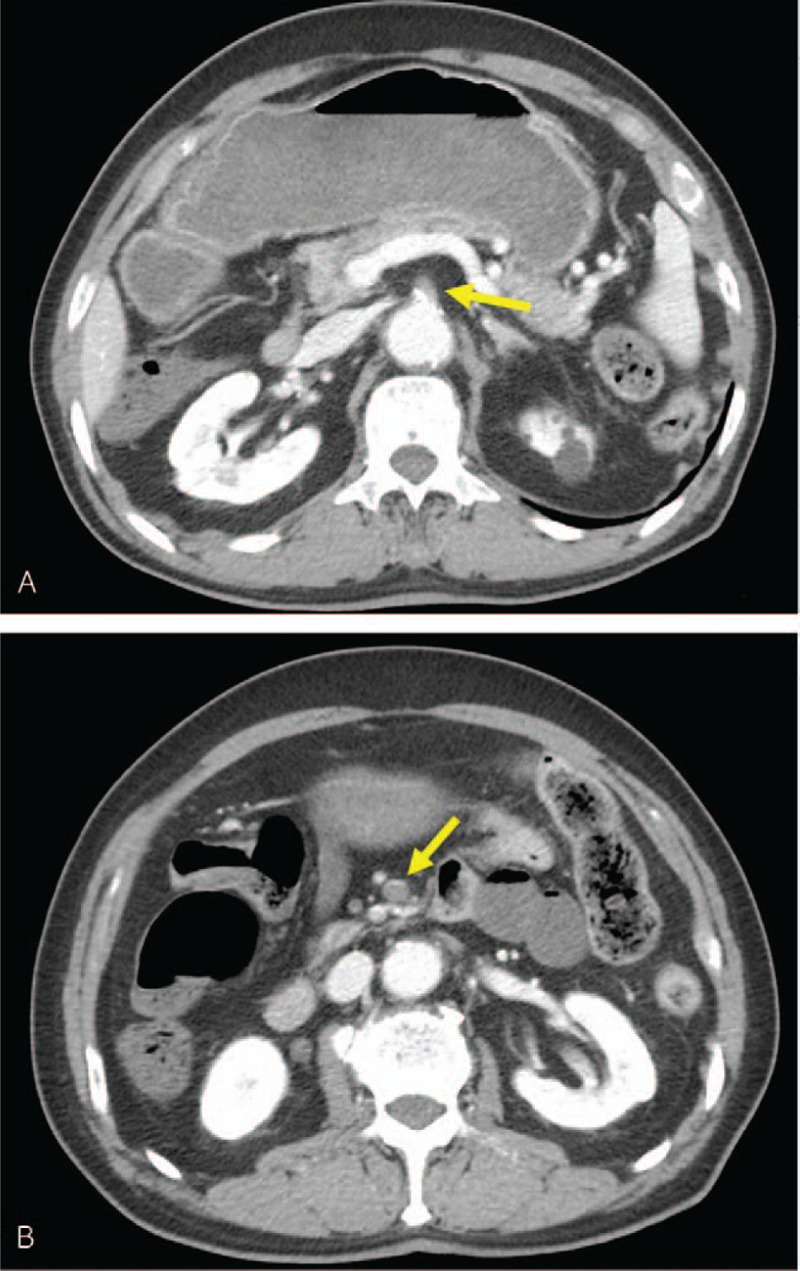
(A) and (B) Abdominal computed tomography scan showing occlusion of the superior mesenteric artery.
